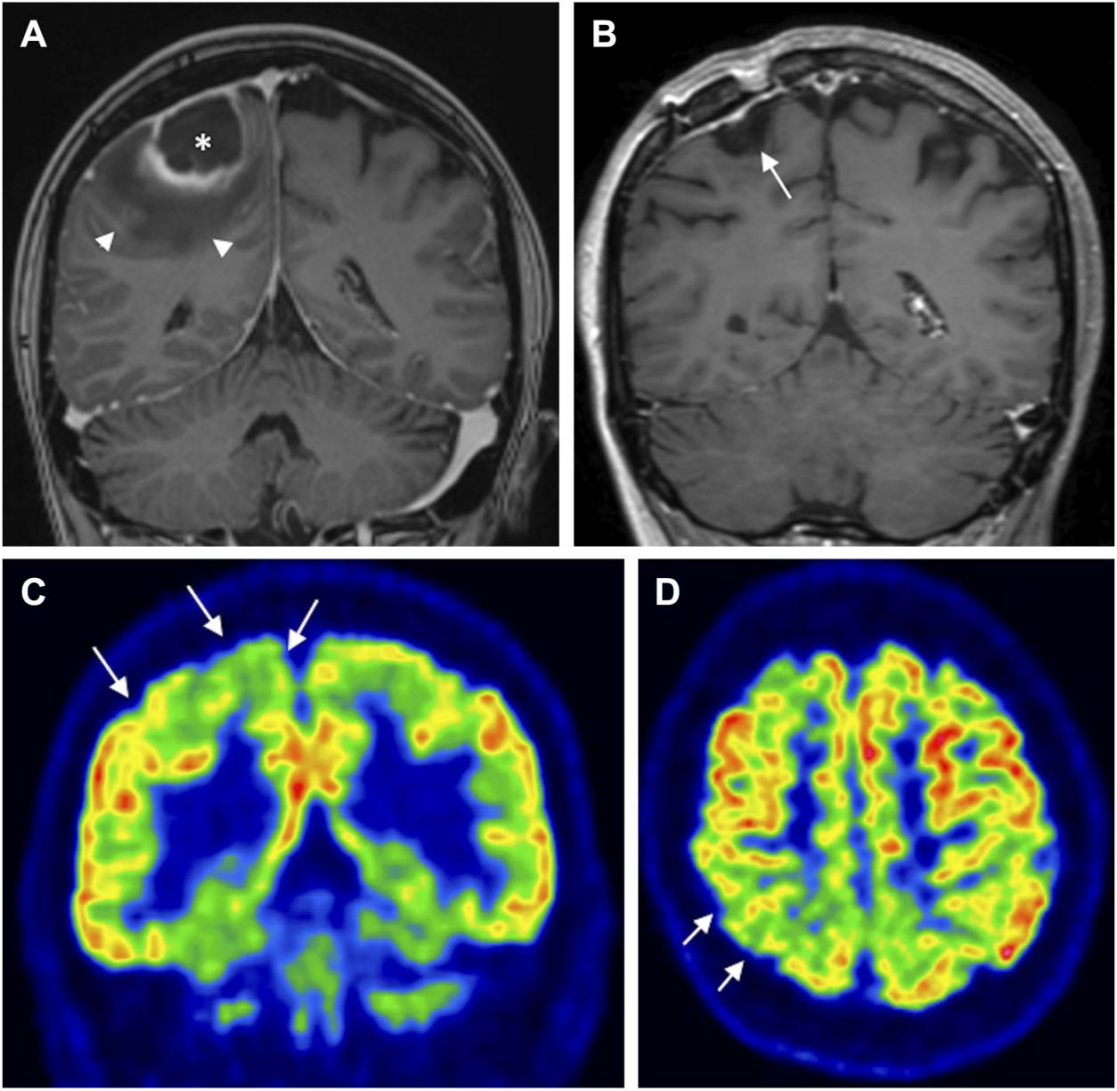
Tissue loss and hypometabolism after brain abscess. A, MRI showing an abscess (*) in the right parietal lobe surrounded by vasogenic edema (arrowheads) before pus removal; B, cortical tissue loss 1 year later (arrow). C and D, [^18^F]-fluoro-2-deoxy-D-glucose positron emission tomography/computed tomography (coronal and axial sections, respectively) in the same patient 3 years after pus removal, showing neocortical hypometabolism (arrows).
